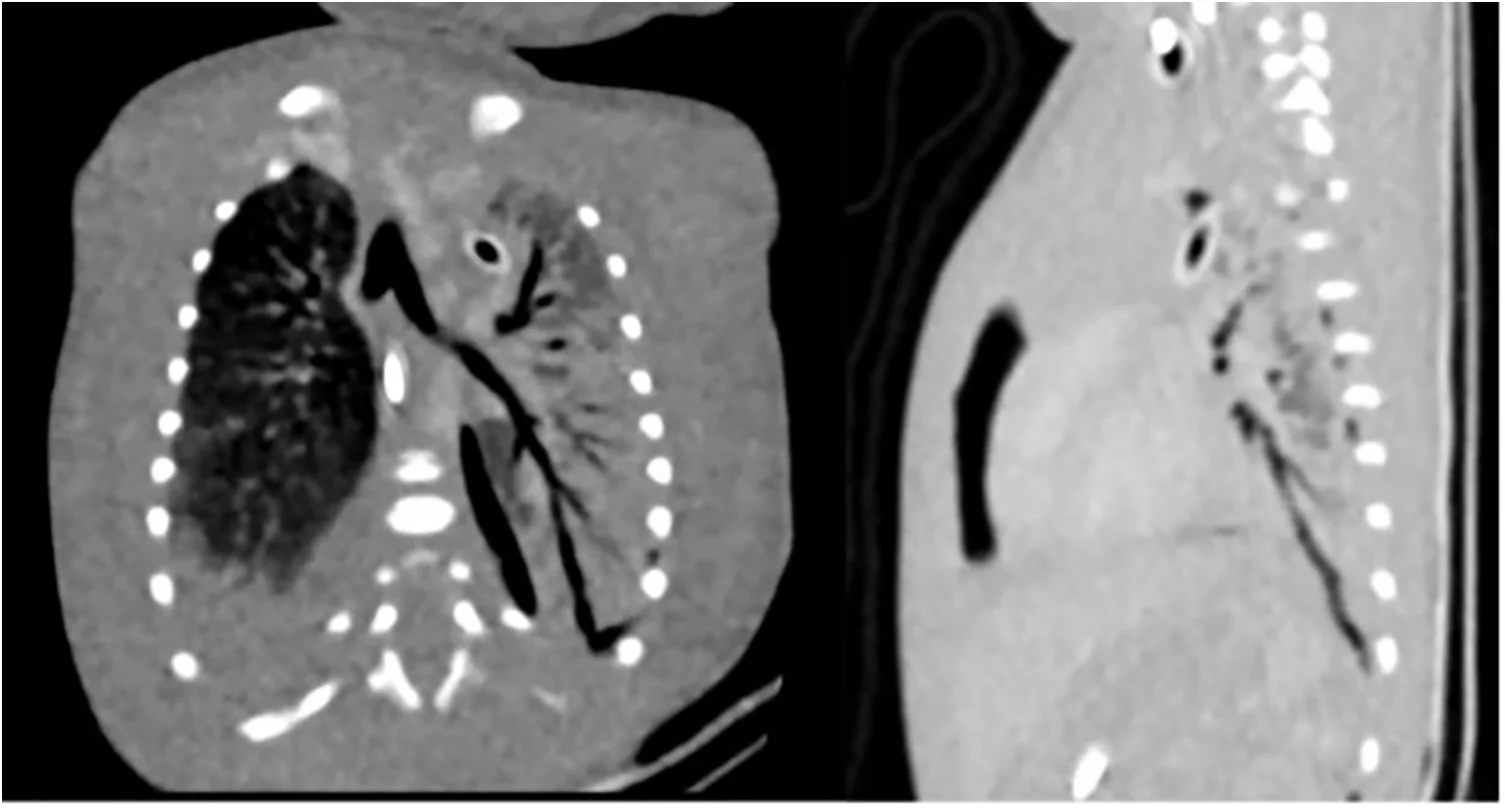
CT scan taken before the procedure (bronchopleural fistula is indicated by the arrow).
